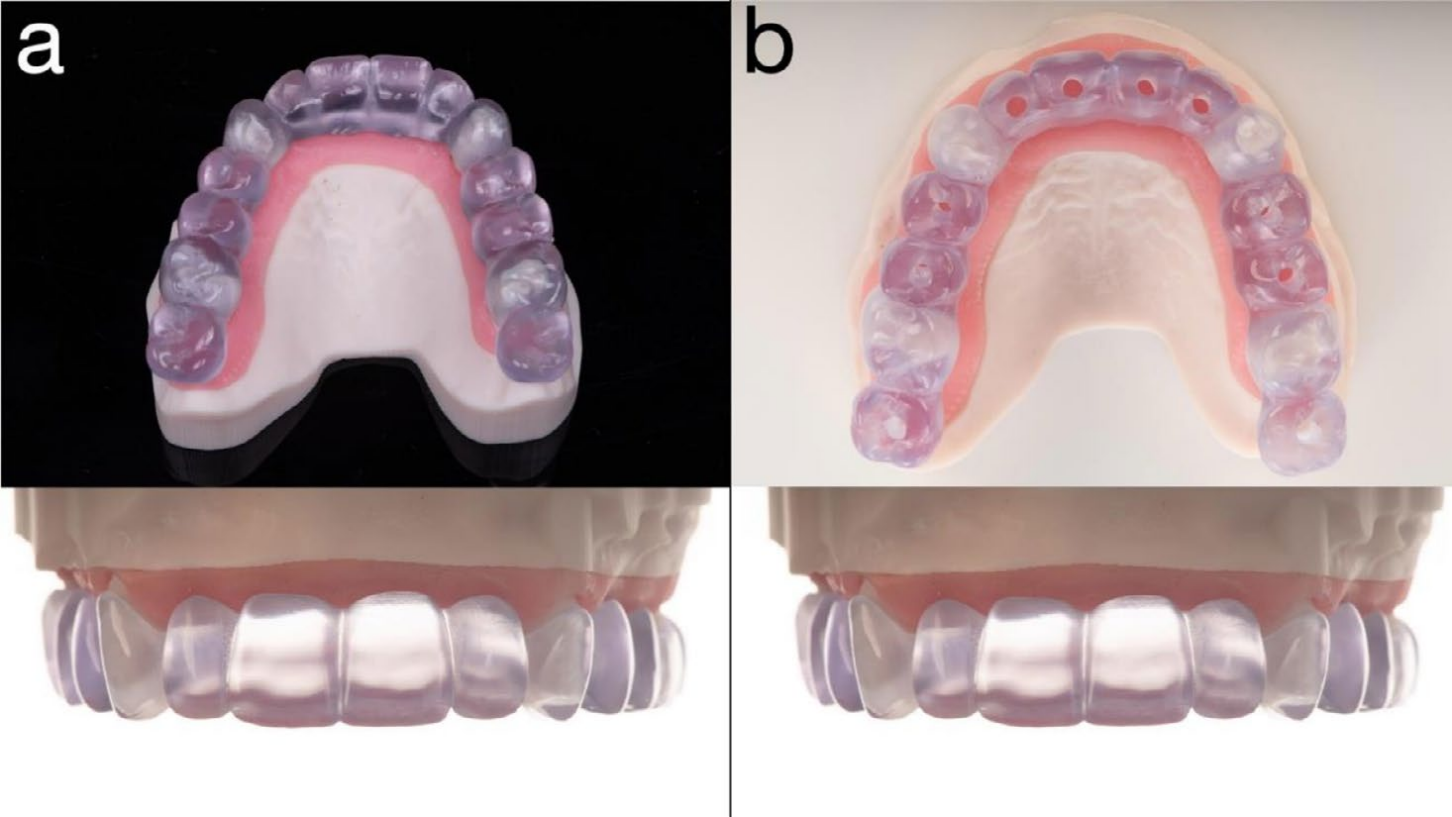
(a) Radiographic stent and (b) surgical stent used for guided implant placement.
